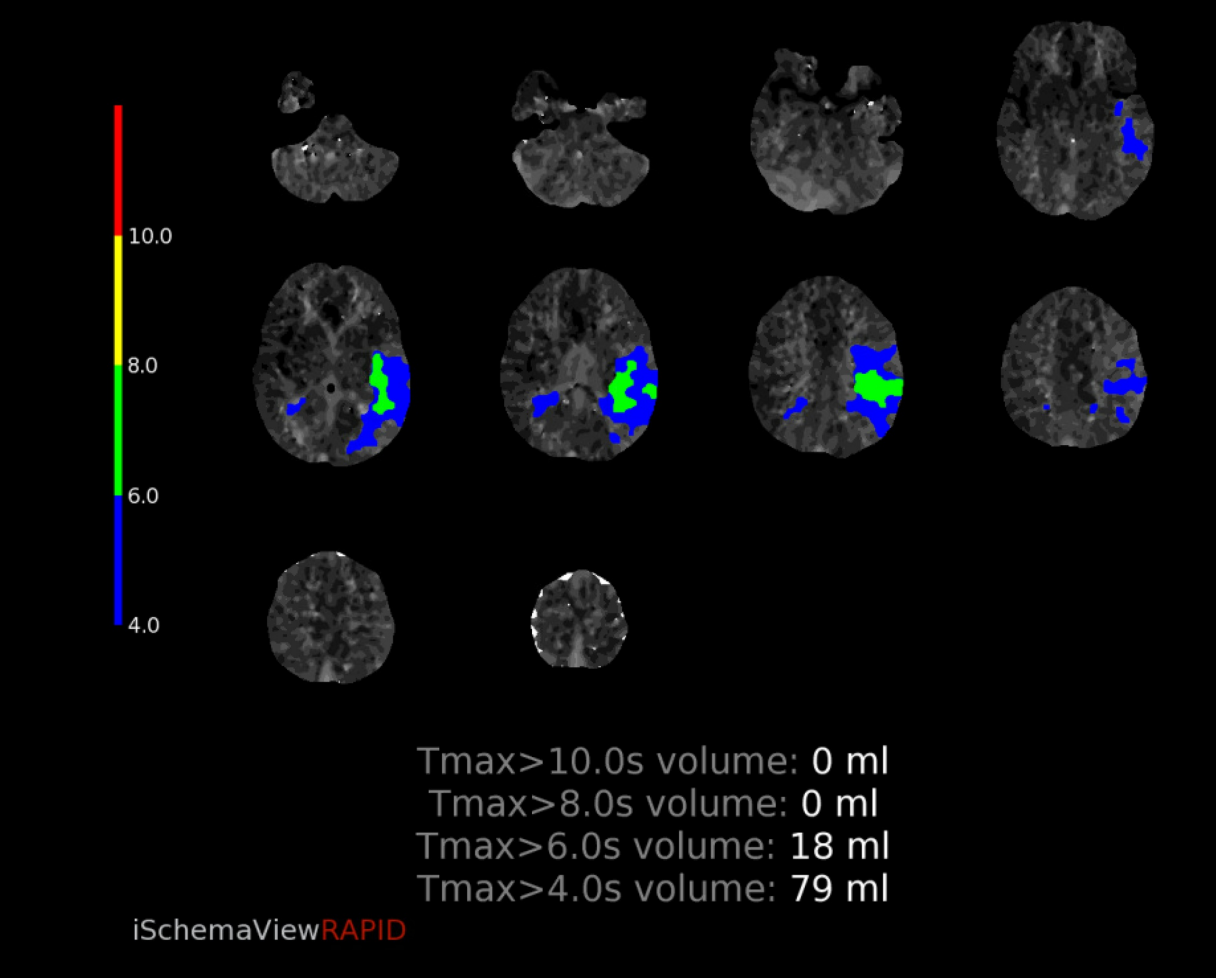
CT perfusion demonstrating elevated Tmax in the left frontal lobe Tmax=Time-to-Maximum
